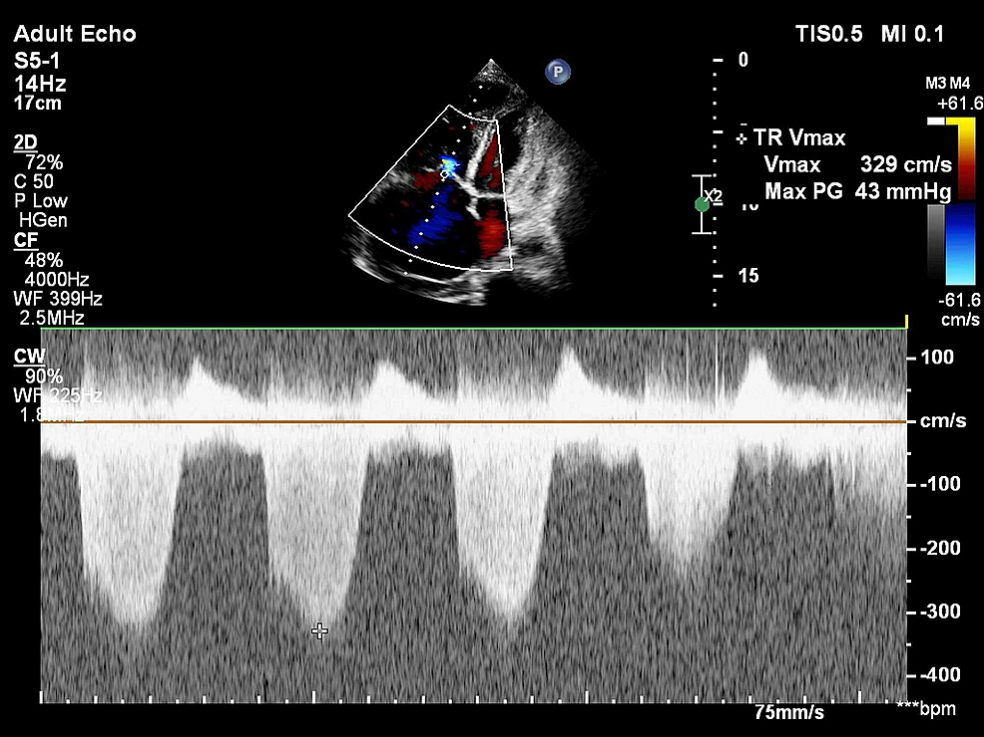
Continuous wave (CW) Doppler signal of the tricuspid valve regurgitation jet
